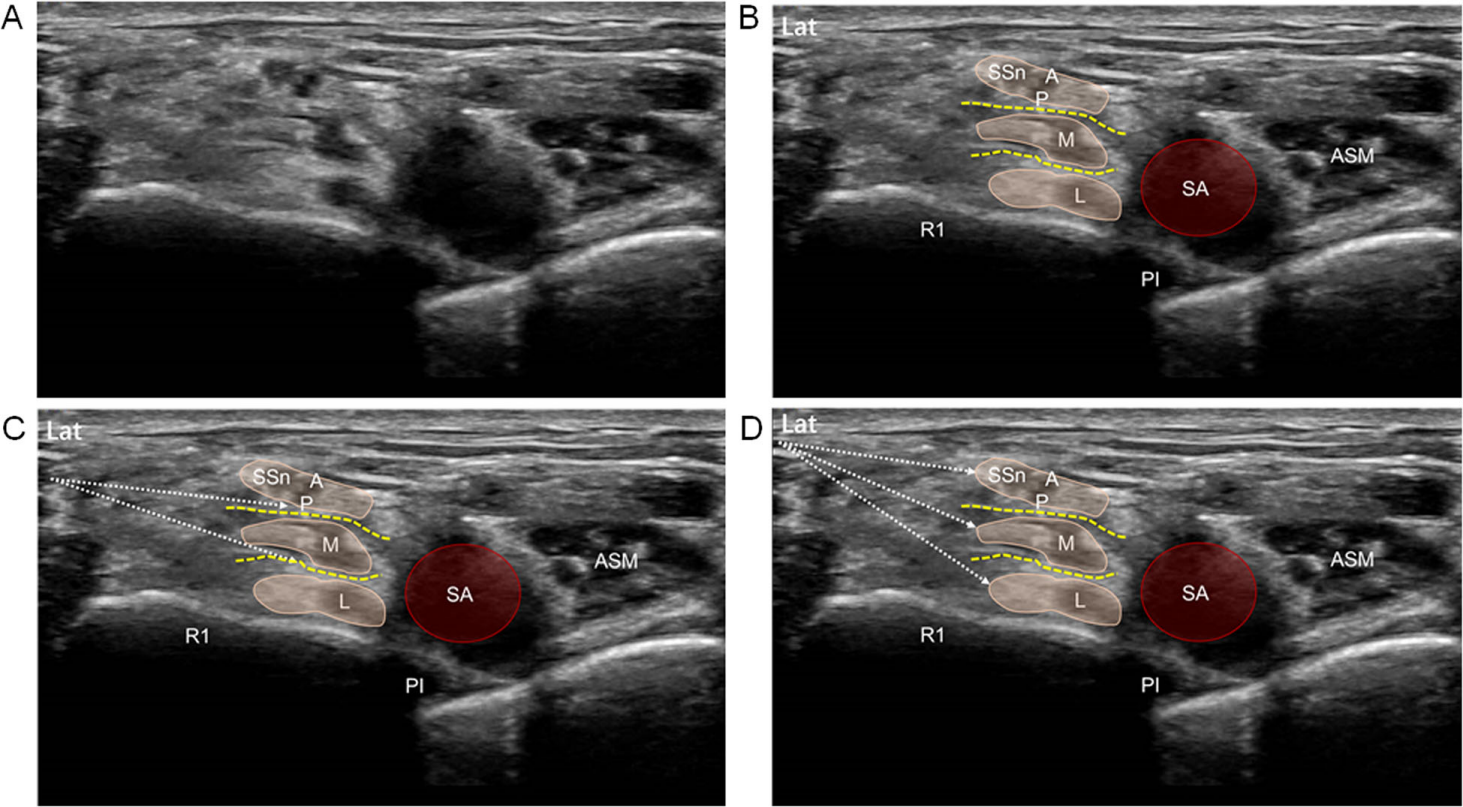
Images demonstrating the satisfactory imaging for IA-SCB (A, B) and the double-injection (C) and triple-injection (D) techniques used in IA-SCB. A, B The satisfactory imaging of the individual trunks of the brachial plexus in the supraclavicular fossa. C Sites of injection in the DI method: the needle tip (dotted arrows) is orientated to the intertruncal planes of the 3 trunks (first injection in the lower intertruncal plane, second in the upper plane), and the desired spread pattern of LA will be confirmed by ultrasound. D Sites of injection in the TI method: the needle tip (dotted arrows) is orientated orderly to the outer boundaries (epineurium) of the trunks, and the desired spread pattern of LA will be confirmed by ultrasound. The injection sequence is from bottom to top, that is the first injection for the lower trunk, second for the middle trunk, and last for the upper trunk. A, anterior division of upper trunk; p, posterior division of upper trunk; SSn, suprascapular nerve; L, lower trunk; M, middle trunk; Pl, pleura; R1, first rib; SA, subclavian artery; dotted line = intertruncal planes; ASM, anterior scalene muscle; LA, local anesthetics; IA, intertruncal approach; SCB, supraclavicular block; DI, double injection; TI, triple injection
